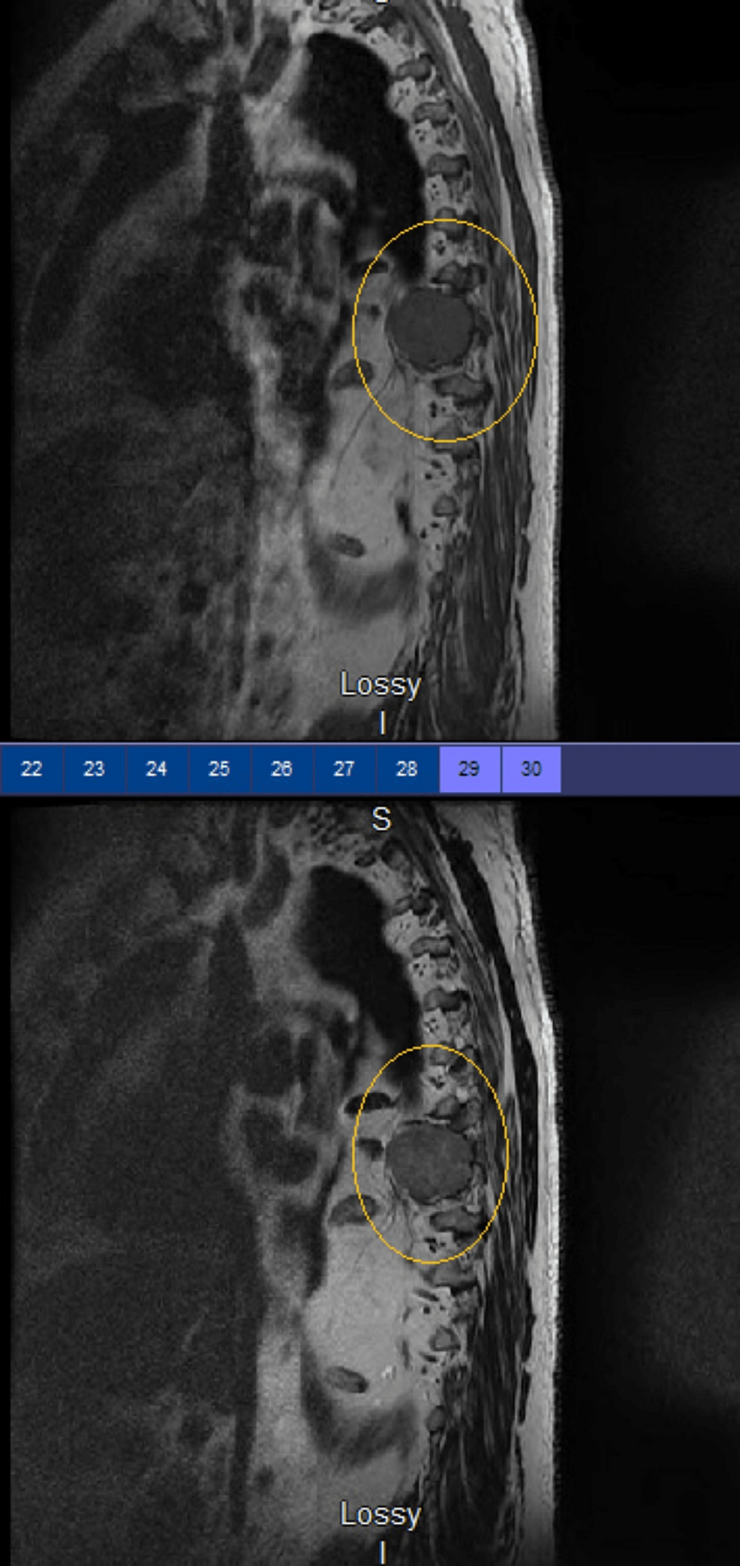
MRI of the thoracic spine with and without contrast revealing a T8-centered infiltrative solid destructive mass lesion with mild spinal cord compression.
